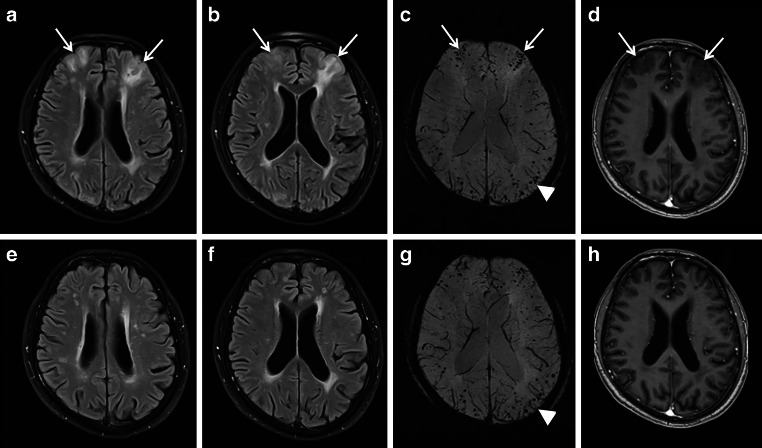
CAA related inflammation (CAA-ri) in a 61-year-old man suffering from subacute psychosyndrome with disturbance of consciousness and executive disorders. a–d bifrontal left dominant hyperintense lesions with sulcal effusions (a,b: fluid attenuated inversion recovery [FLAIR] images ax.; *arrows*), accentuated microbleeds (MB) (c, susceptibility-weighted imaging [SWI] ax.; *arrows*) and hypointense signal conversion on postcontrast (pc) T1 WI (d, *arrows*) with enhancement; e–h follow-up MRI 13 months later after 3 bouts of high-dose methylprednisolone infusions, neurological examination was unremarkable. Completely resolved lesions frontal (e,f), unchanged cortical and subcortical MB (c,g; *arrowheads*)
